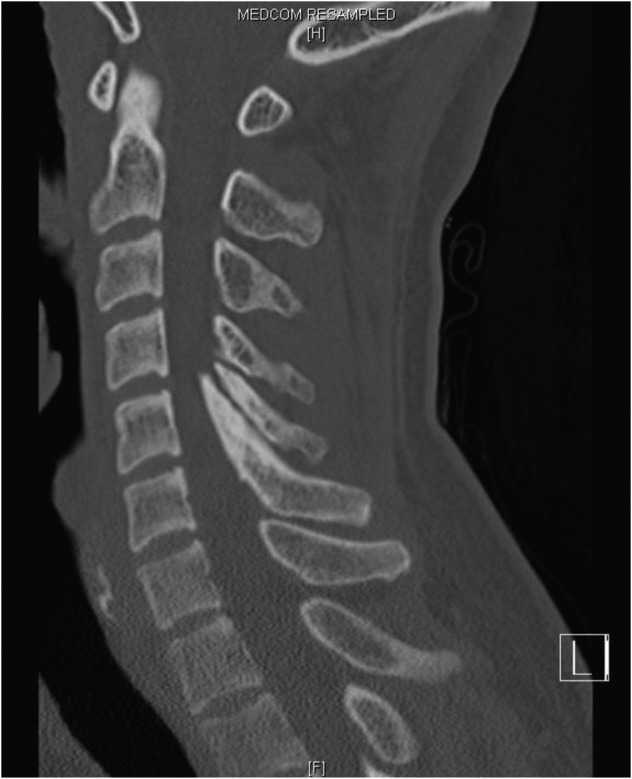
Sagittal computed tomography image of the cervical spine showing a beak like outgrowth from the posterior elements protruding into the spinal canal, the classical appearance of an ossified yellow ligament.
